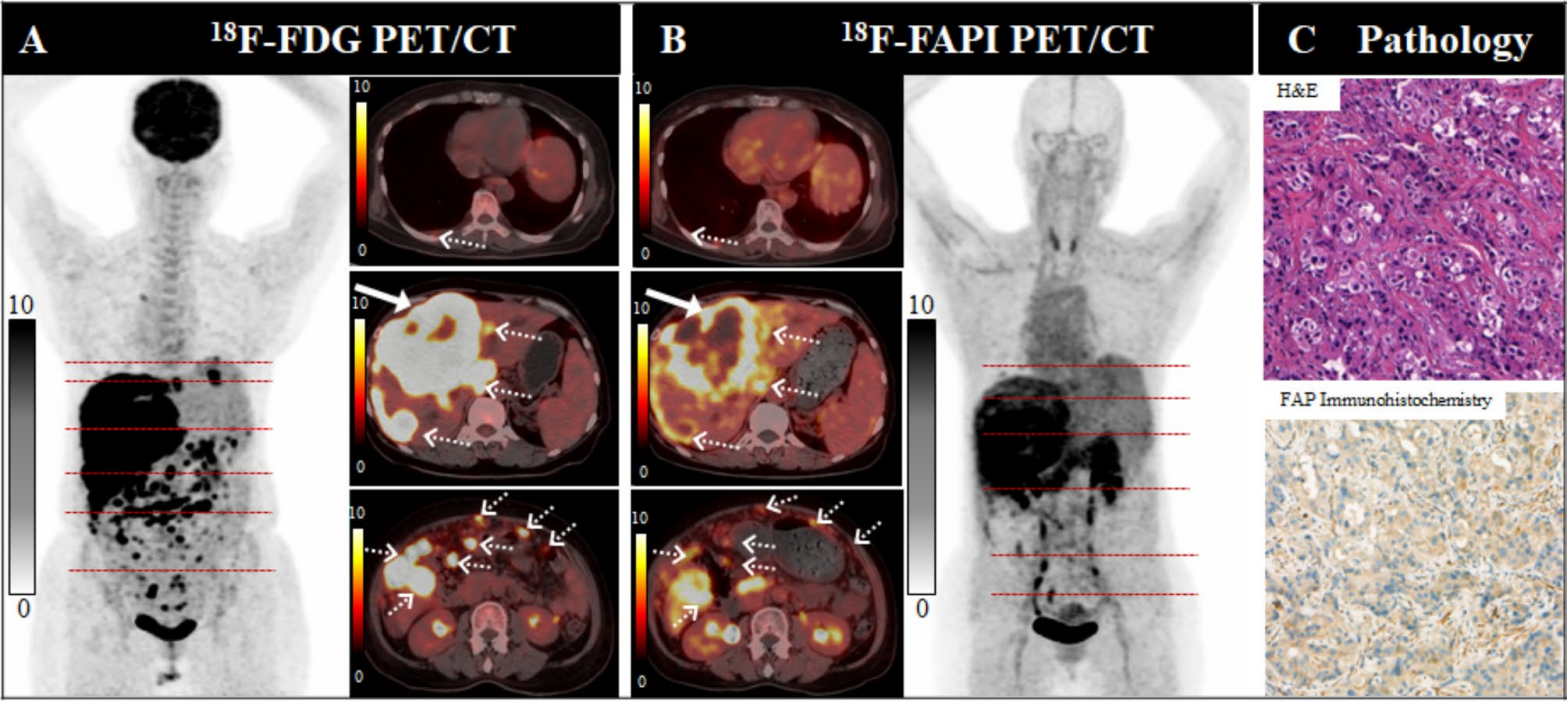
A 61-year-old female patient (Patient No. 22) with ICC was confirmed by biopsy. [^18^F]FDG PET/CT (A) demonstrated higher radiotracer uptake in the primary tumor (solid arrow), intrahepatic subfoci, lymph node and peritoneal metastatic lesions (dotted arrows), compared with [^18^F]FAPI-04 PET/CT (B). The pleural metastases exhibited a comparable radiotracer uptake to FAPI PET/CT (white arrows). Pathological findings (C) indicate a heightened expression for FAP
